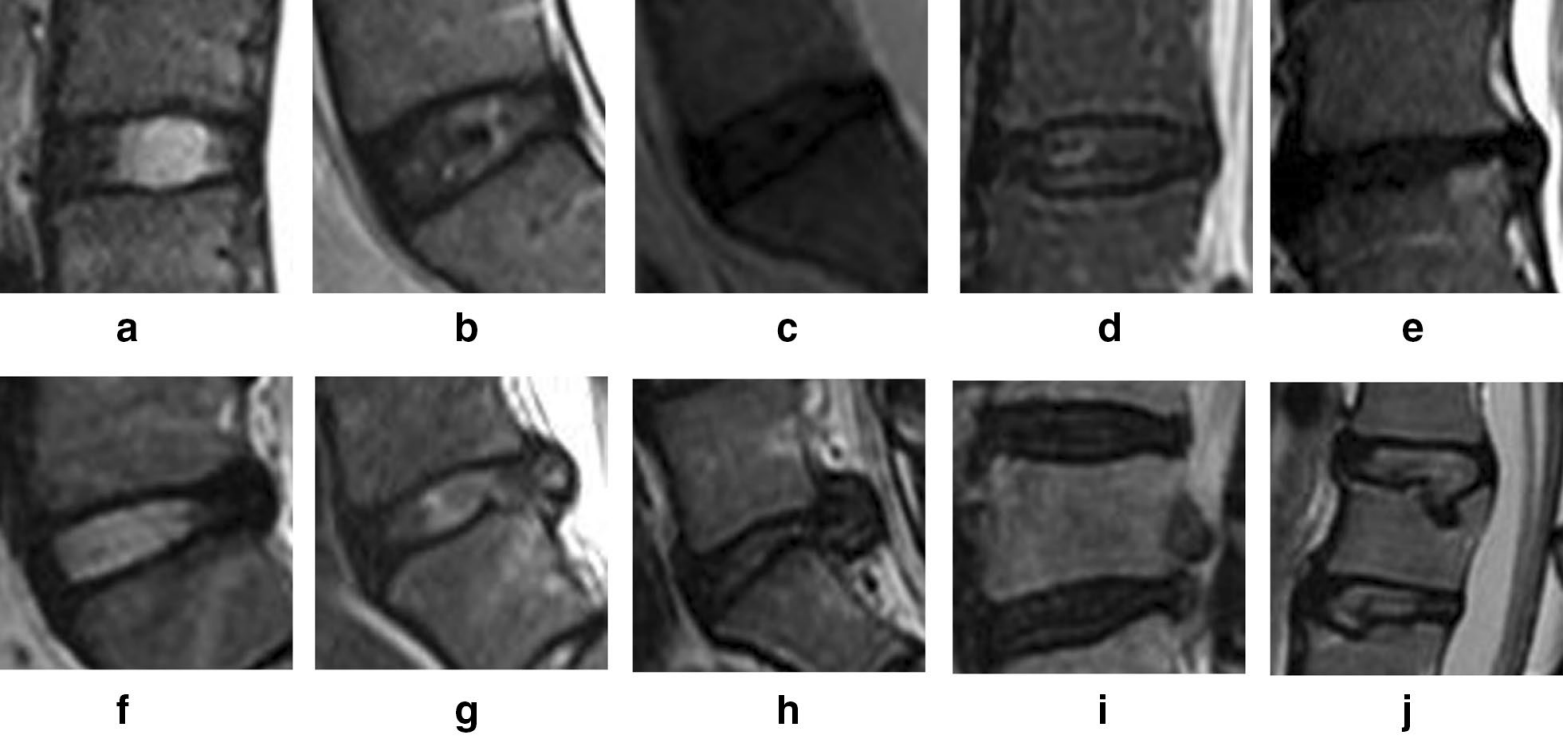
Mid-sagittal view on T2-weighted images of the Lumbardiscs. a (Normal), b (Blurred disc as early stage of LDD), c (Black disc), d (Black + bulging disc), e (Decreased height + protrusion disc), f (Pure bulging disc), g (Pure protrusion), h (Extrusion), i (sequestration), j (Schmorl node, SN)
